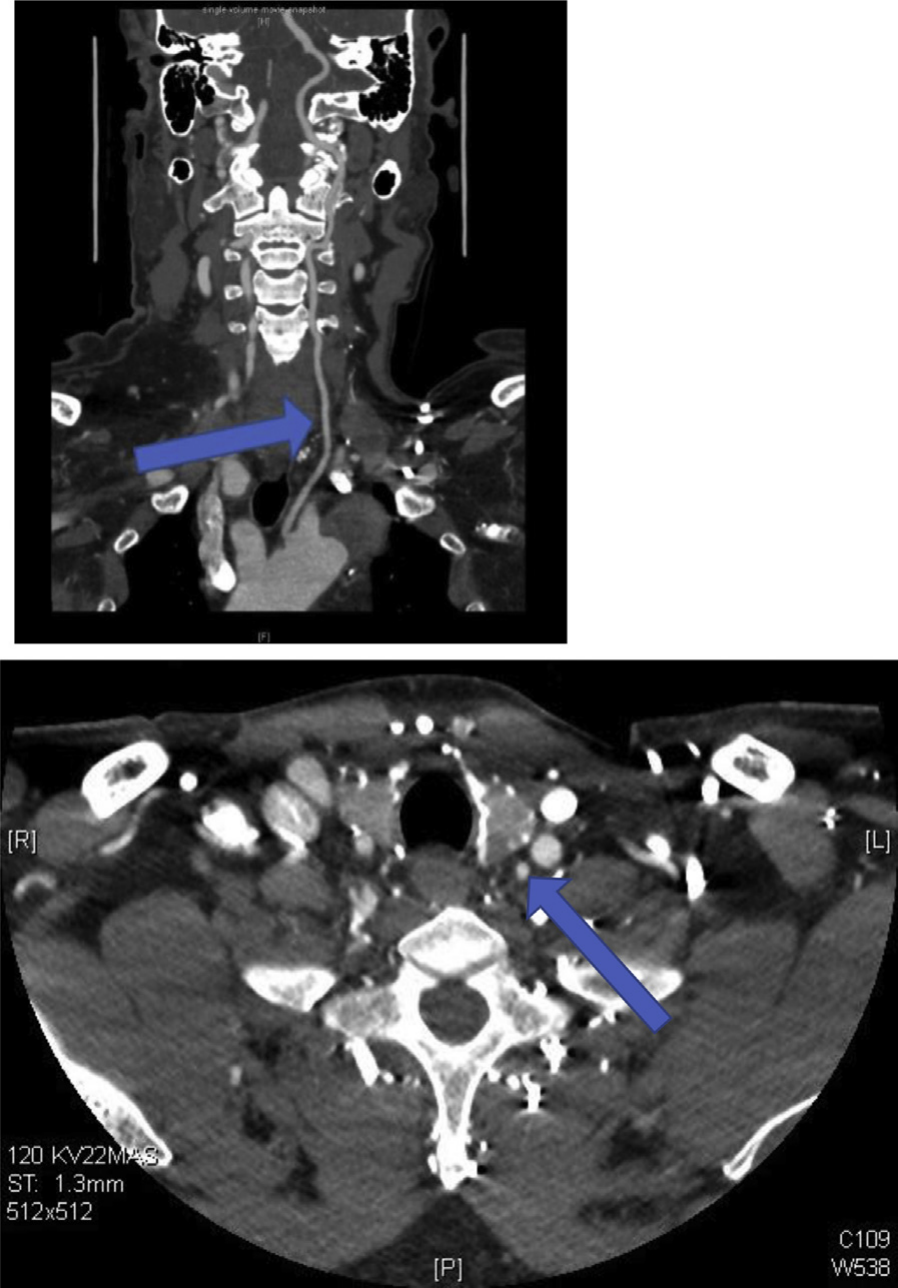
Follow-up computed tomography (CT) scans after 5 months showing left vertebral artery aberrantly arising from the aortic arch (*blue arrow*; Top) and the left vertebral artery coursing posteriorly in the left carotid sheath (*blue arrow*) before entering the C6 transverse foramen (Bottom).
